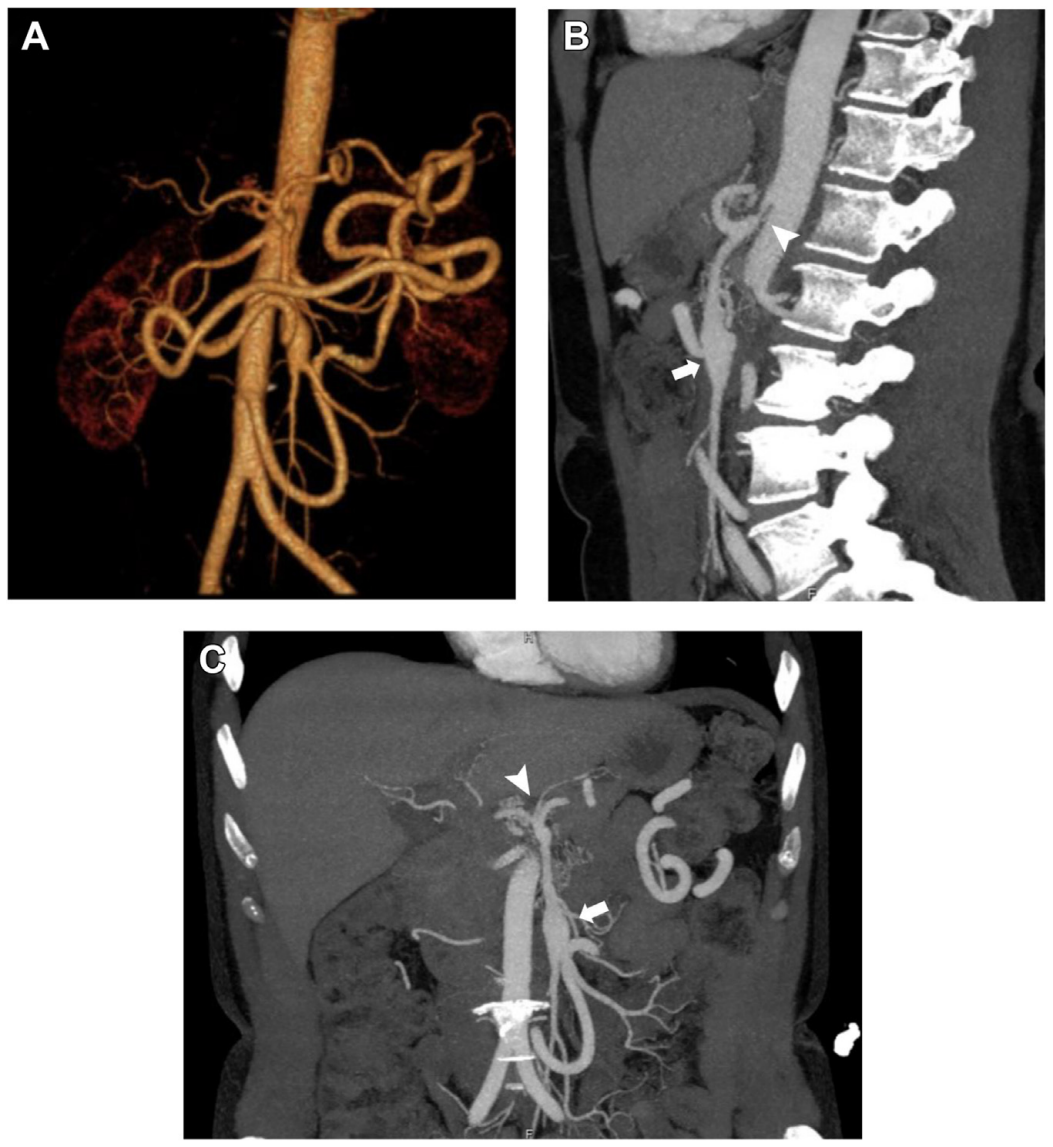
Preoperative computed tomography angiograms. A, Three-dimensional reformatted computed tomography angiogram demonstrating a celiacomesenteric trunk (CMT), large inferior mesenteric artery collateral vessel, and a 1.8-cm superior mesenteric artery (SMA) aneurysm. B, Sagittal computed tomography angiogram cut depicting origin of the CMT with compression (*white arrowhead*) and SMA aneurysm (*white arrow*). C, Coronal computed tomography angiogram cut depicting CMT (*white arrowhead*) and SMA aneurysm (*white arrow*).
